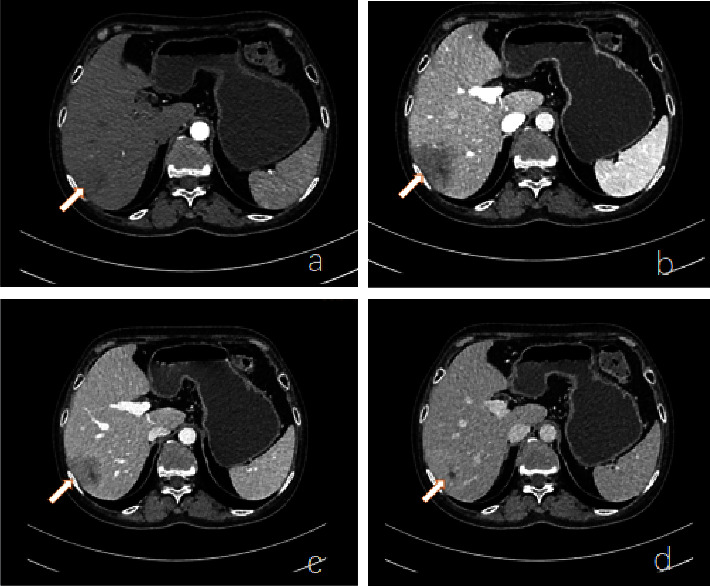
Abdominal enhanced CT on day 16 of admission. On the 16th day of admission, abdominal enhanced CT displayed that the size of the abscess in the right lobe of the liver was 3.4 cm, which was smaller than before. Arterial phase (a); venous phase (b); equilibrium phase (c); delay phase (d).
